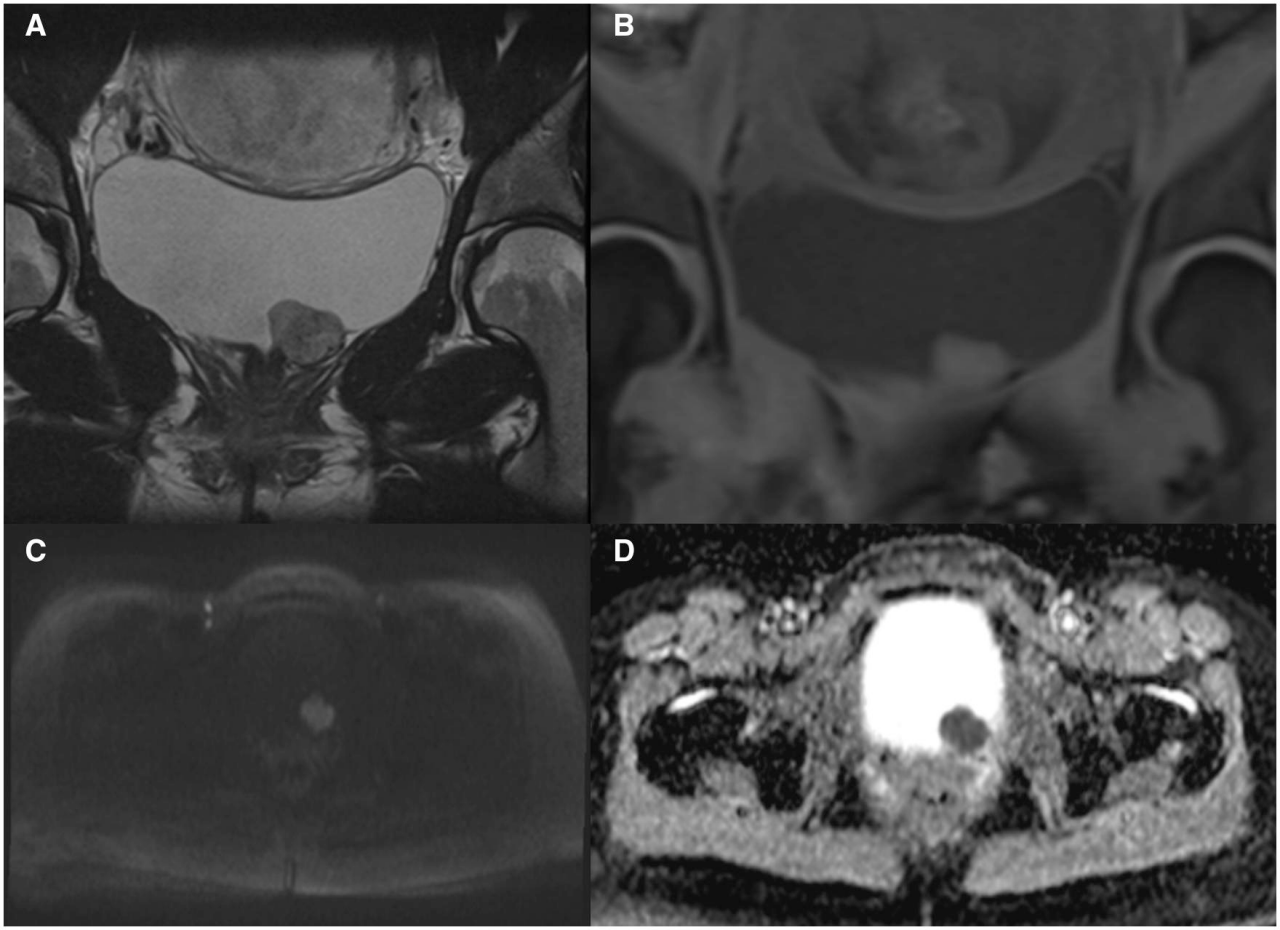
(A) A T2 image showing a well-defined lesion from the anterior bladder wall with homogenous T2 intermediate signal changes and hypointense foci representing internal vascularity, with (B) corresponding to the coronal view of the mass in T1 with mild hyperintensity. (C) An axial view in the DWI sequence showcasing the restricted diffusion of the mass. (D) The same lesion with hypointense signal on ADC map, further suggesting restricted diffusion. Abbreviations: ADC = apparent diffusion coefficient; DWI = diffusion-weighted image. Images were taken from the Gold Coast PACS system.
